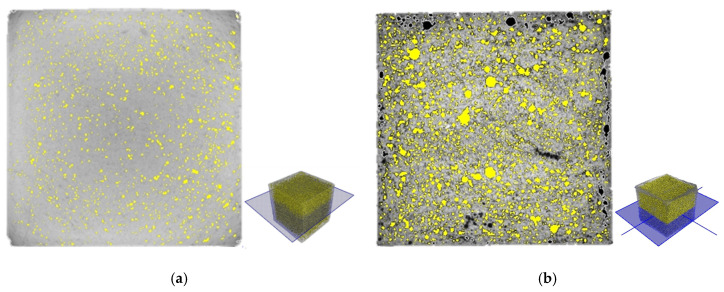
Cross-sectional images of (a) PCM1-1 and (b) PCM3-3 from the X-ray CT analysis.
